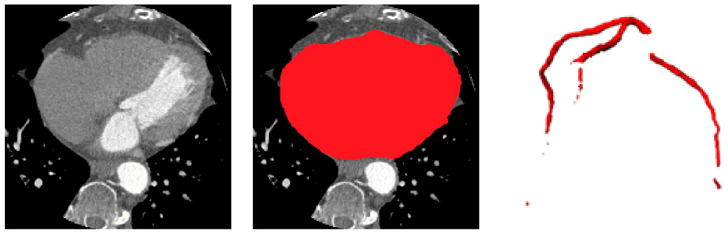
Heart and Coronary Vessel Segmentation: original CT slice, predicted heart region of interest (ROI) and 3D coronary artery segmentations.
